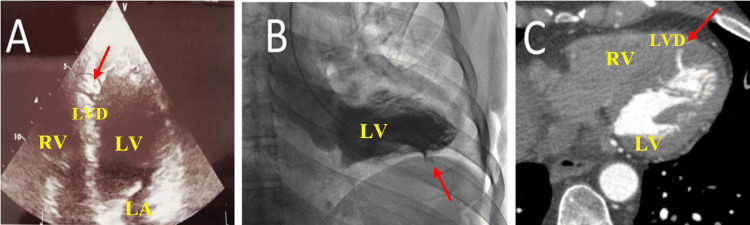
A: Apical four-chamber view showing left ventricular diverticulum. B: Left ventricular ventriculogram showing left ventricular diverticulum. C: Cardiac angiotomography showing left ventricular diverticulum LV=left ventricle; LA=left auricle; RV=right ventricle; LVD=left ventricular diverticulum; Red arrows=pointing LVD
